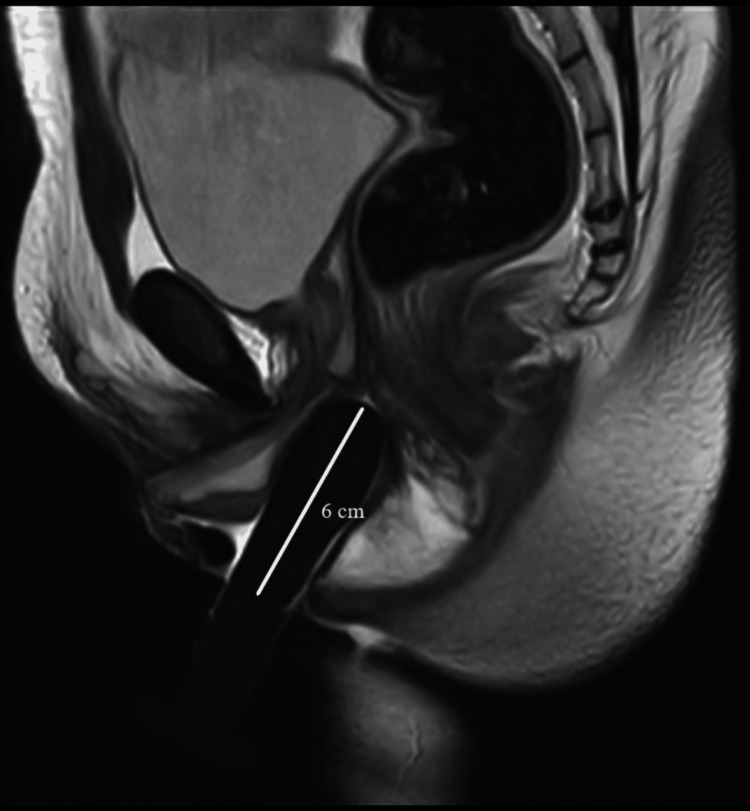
T2W HR sagittal image of defaulter patients Original MRI image of a patient who underwent the study.
